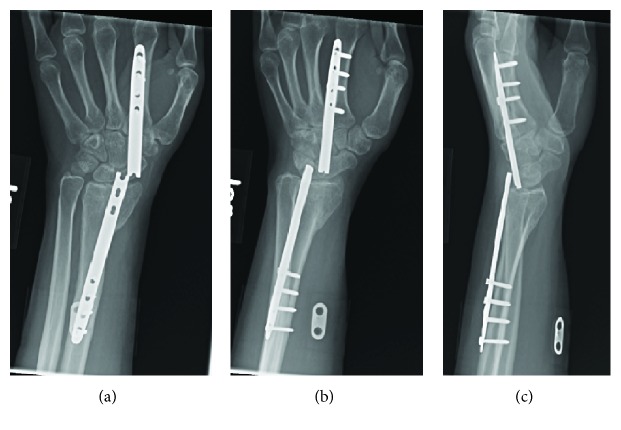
Return to clinic. X-rays on return to clinic, 12.5 months after dorsal bridge plate placement.
